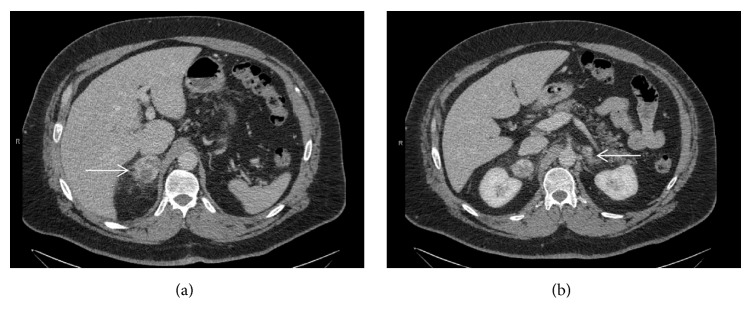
CT scan of the abdomen showing the right adrenal tumor ((a), arrow) and the left adrenal tumor ((b), arrow) prior to adrenalectomy.
